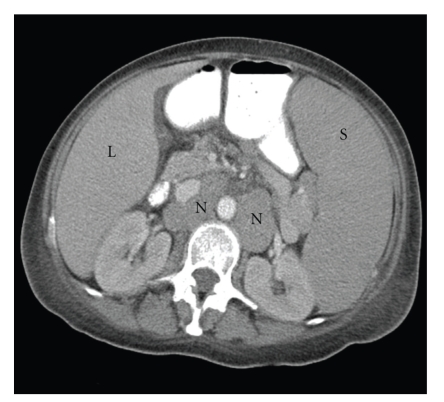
Computed tomography scan of the abdomen showed massive splenomegaly and extensive lymphadenopathy . (L: liver, S: spleen, N: lymphadenopathy).
